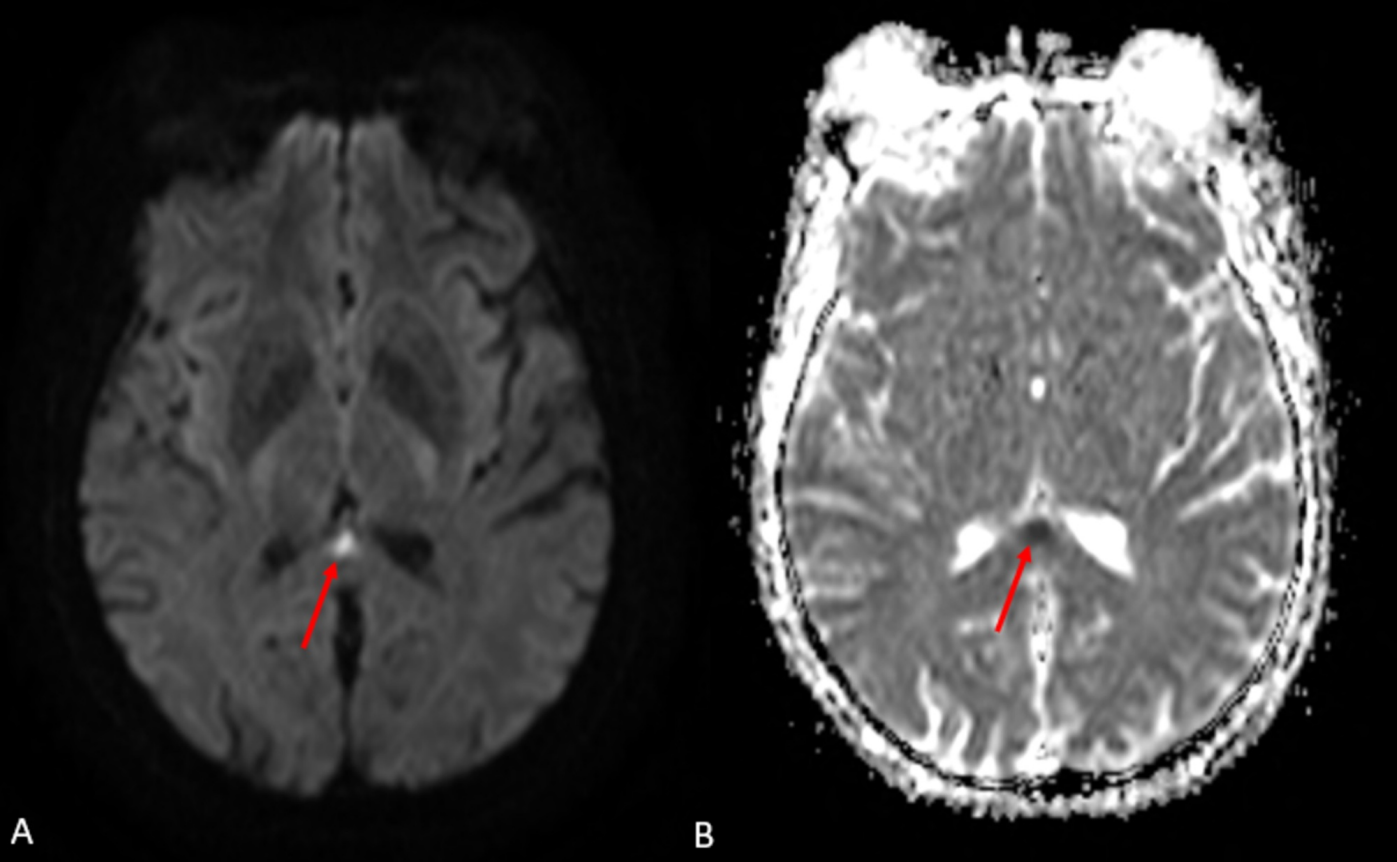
Axial MRI with (A) diffusion-weighted imaging (DWI) sequence and (B) apparent diffusion coefficient (ADC) sequence The images show increased signal within the splenium of the corpus callosum on the DWI (A) with corresponding signal dropout on the ADC (B) (red arrow). This finding may be secondary to metabolic derangement, focal infarct, or infection MRI: magnetic resonance imaging
